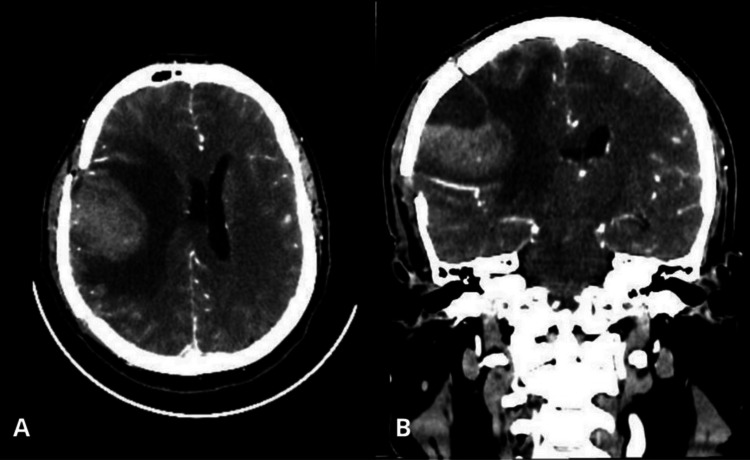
Contrast-enhanced computed tomography in the arterial phase of the skull A: contrast-enhanced computed tomography in the arterial phase of the axial skull showing the cerebral parenchyma with nodular solid imaging at the lobe level; B: contrast-enhanced computed tomography in the arterial phase of the coronal skull showing the cerebral parenchyma with nodular solid imaging at the lobe level.
